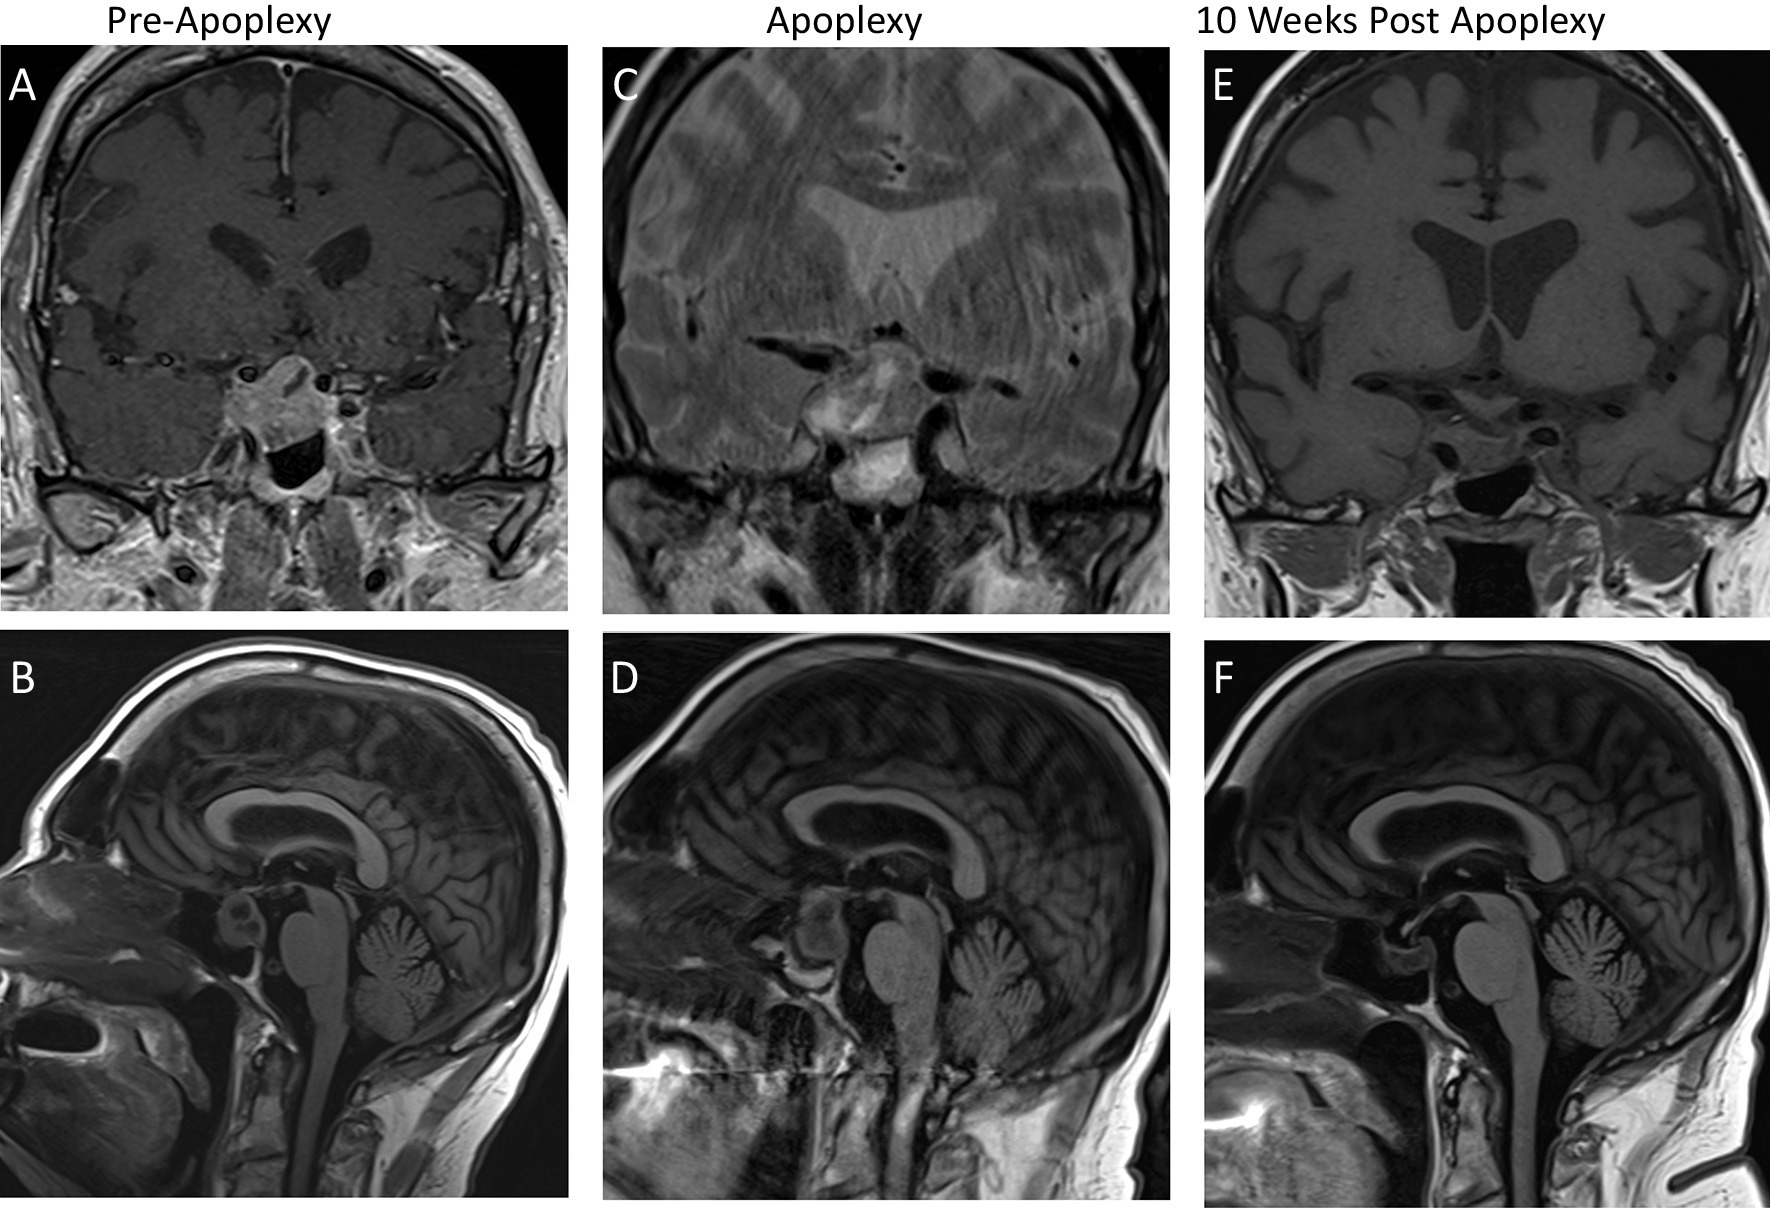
Preoperative MRI shows pituitary macroadenoma (2.7 cm) with suprasellar extension and compression of optic chiasm, parasellar extension into right cavernous sinus, and extension into right sphenoid sinus on coronal T1 with contrast (a) and sagittal T2 FLAIR (b). Postoperative day 7 MRI demonstrates hemorrhage on coronal T2 (c) and sagittal T2 FLAIR (d). Postoperative week 10 MRI demonstrating resolution of hemorrhagic changes within pituitary gland, decrease in size of adenoma now limited to sella, and no mass effect on optic chiasm on coronal T1 without contrast (e) and sagittal T2 FLAIR (f)
